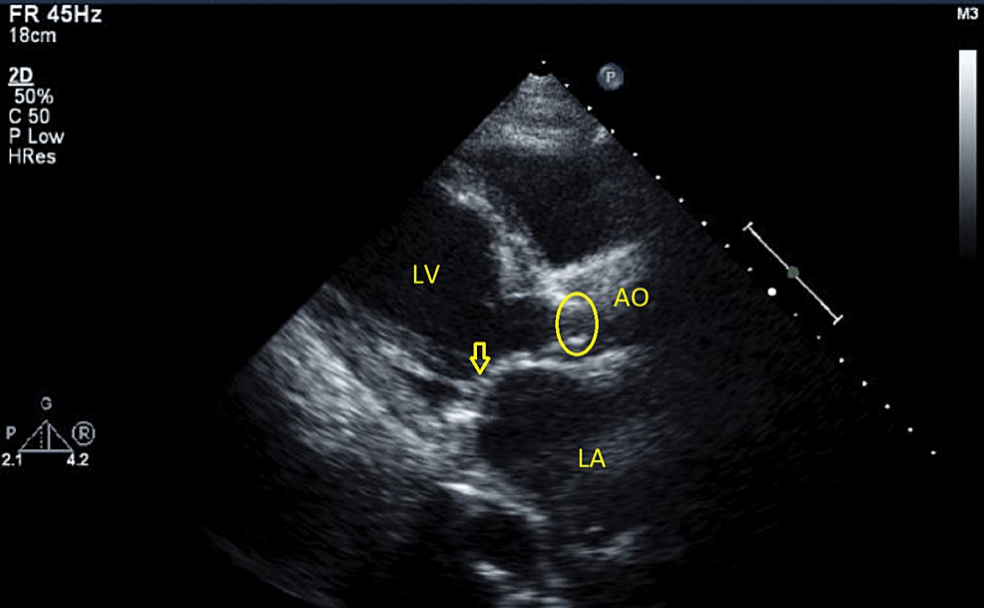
2D ECHO showing closed MV and open AV 2D ECHO: two-dimensional echocardiography; MV: mitral valve; AV: aortic valve; LA: left atrium; LV: left ventricle.
